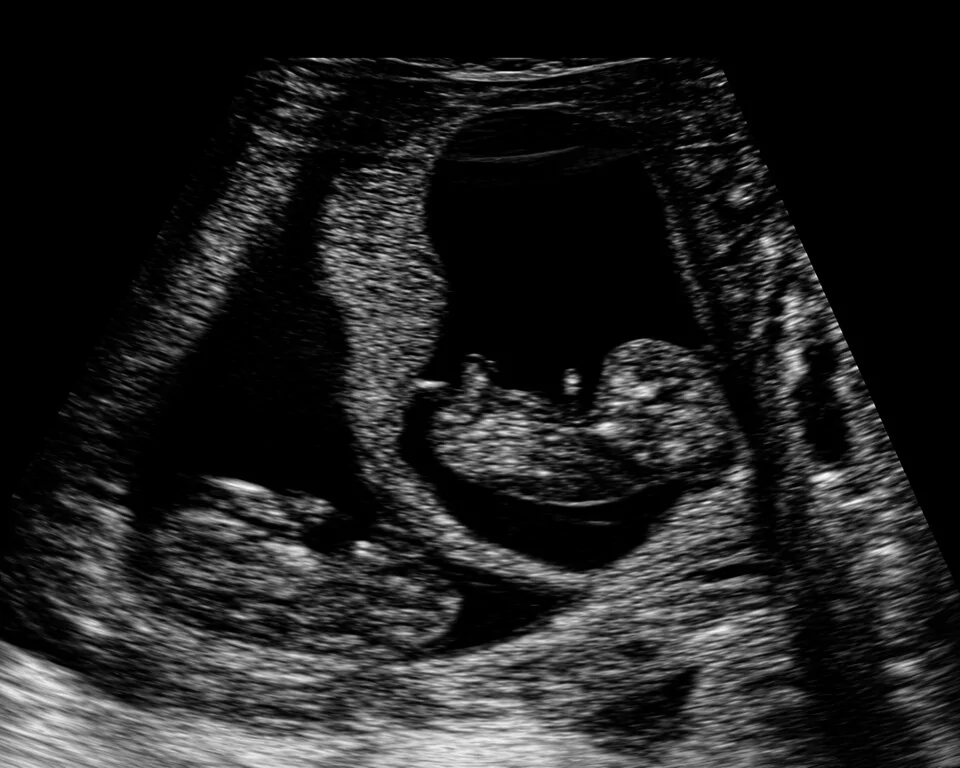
First-trimester ultrasound demonstrating a twin intrauterine pregnancy with a thick dividing membrane and probable twin-peak sign. No angular or cornual implantation identified.
